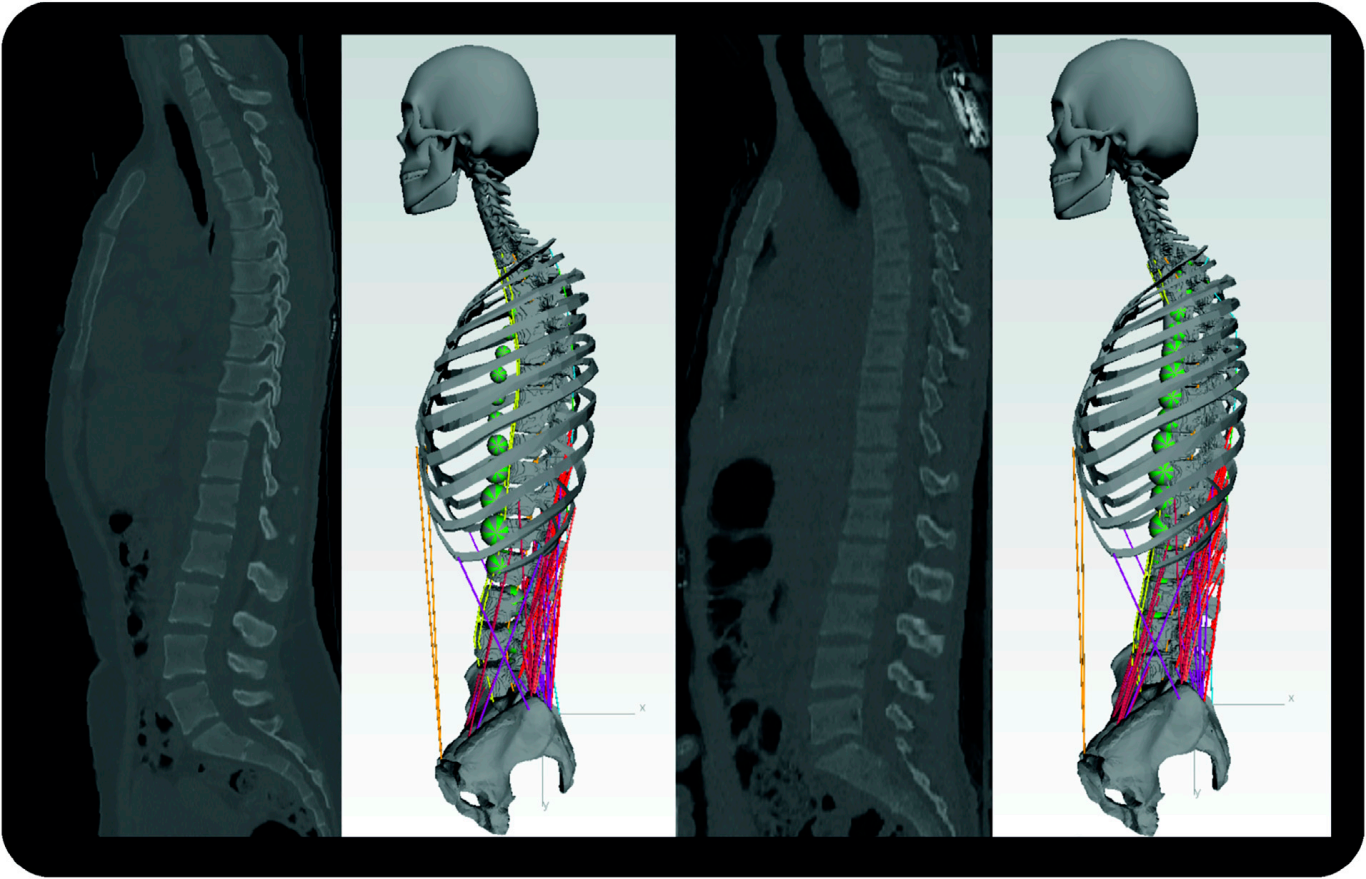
Sagittal CT images of subject one (left) and subject two with respective MBS models. Segment masses for soft tissues are visualized by the green spheres. For the sake of clarity, the dummy bodies for the arms are not shown here.
